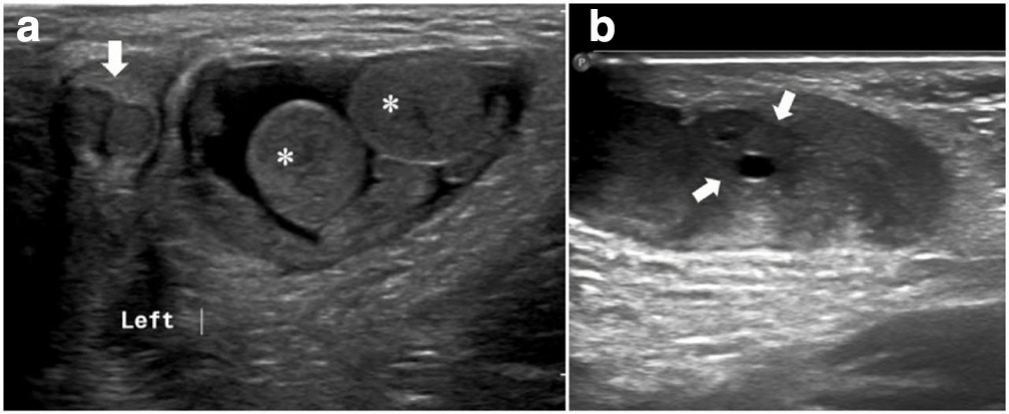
(a) Grayscale ultrasound images. A hernial sac containing two testicles (asterisk) and a partially scanned phallus with two corpora cavernosum (arrow). (b) An ovary containing small peripheral follicles (arrows).
